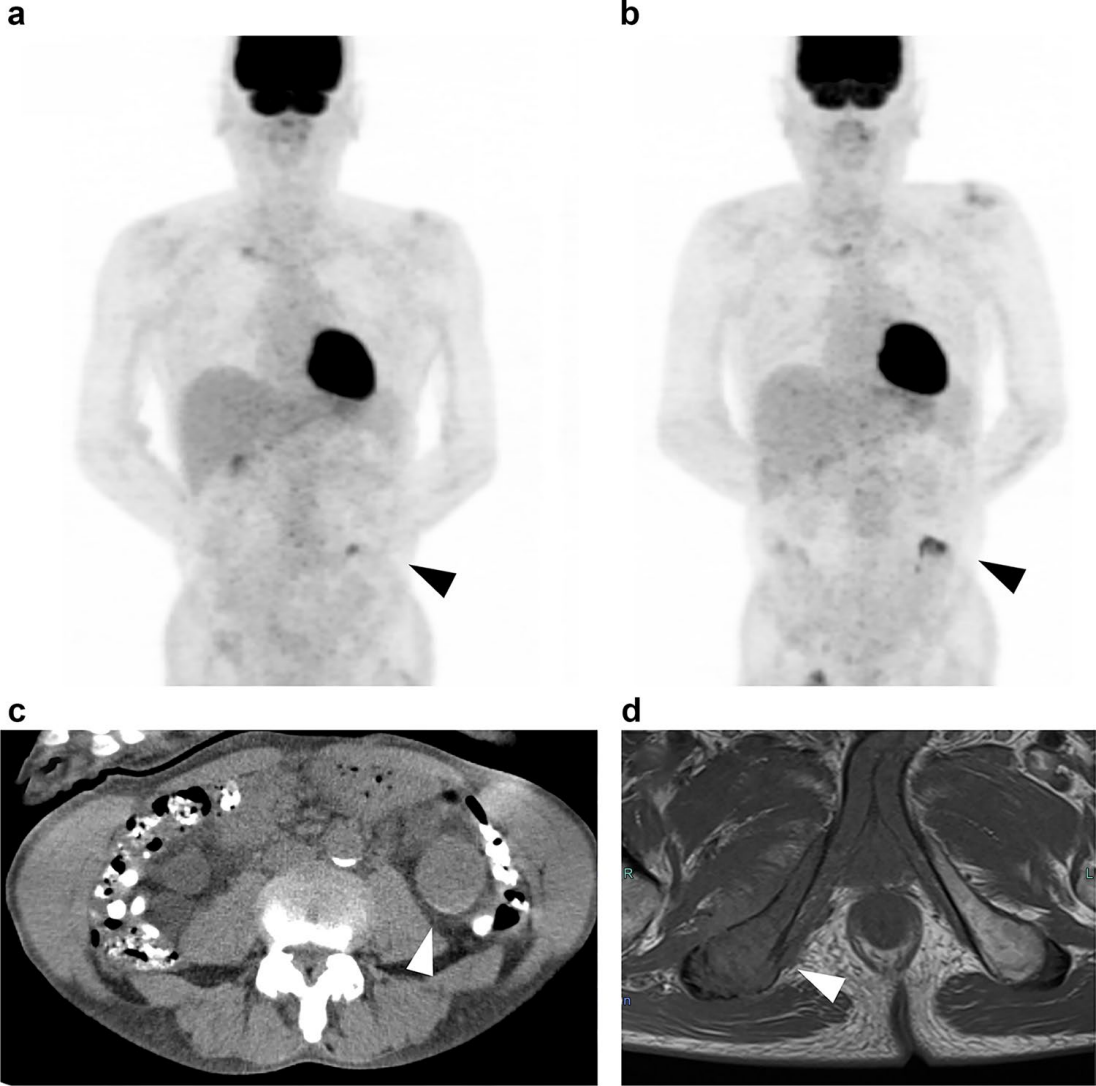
67-year-old man with pathologically proven RCC metastasis in the right ischial bone. a FDG-PET: Maximum intensity projection (MIP) image of initial FDG-PET/CT study shows a focal accumulation with an SUV_max_ of 4.0 consistent with the inferior pole of the left kidney (arrow). b FDG-PET (MIP image) 27 months after the initial investigation shows the accumulation consistent with the left kidney has increased in size, with an SUV_max_ of 5.0 (arrow). c Non-contrast-enhanced CT at the time of study participation had no findings suggesting malignancy. d Axial T1-weighted image of the pelvis 27 months after study participation depicted a hypointense lesion in the right ischial bone (arrow). This lesion was biopsied, and the pathological diagnosis was found to be consistent with RCC metastasis
